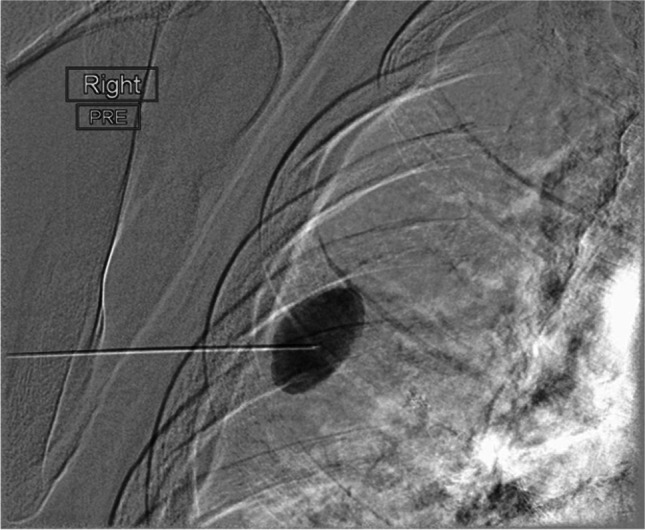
The intercostal pseudoaneurysm section is percutaneously embolized with 3.5 ml of thrombin directly in the lumen under local anesthetic in the computerized tomography (CT) suite
